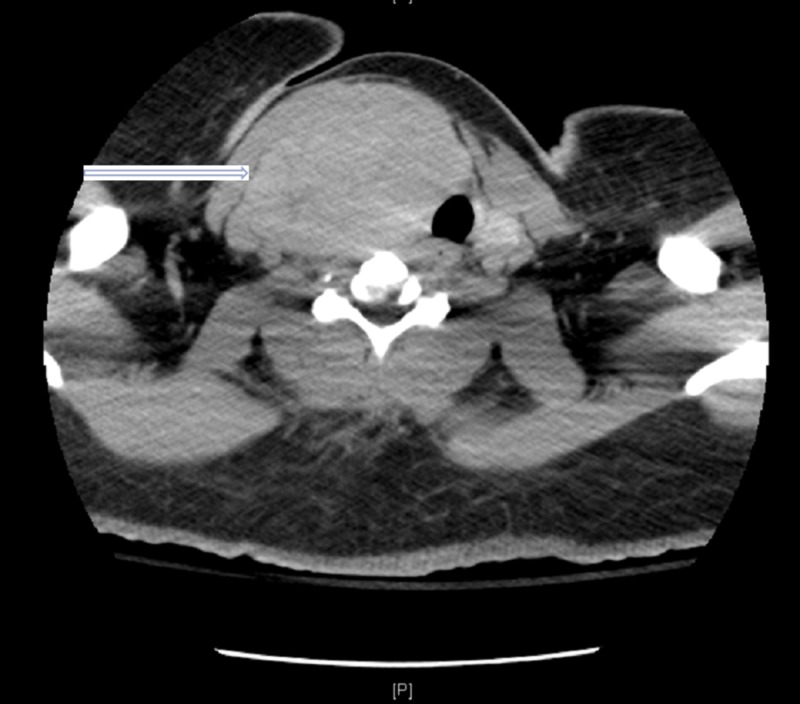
Axial view of CT neck with intravenous contrast showing right thyroid mass (white arrow) with tracheal deviation CT: computed tomography
